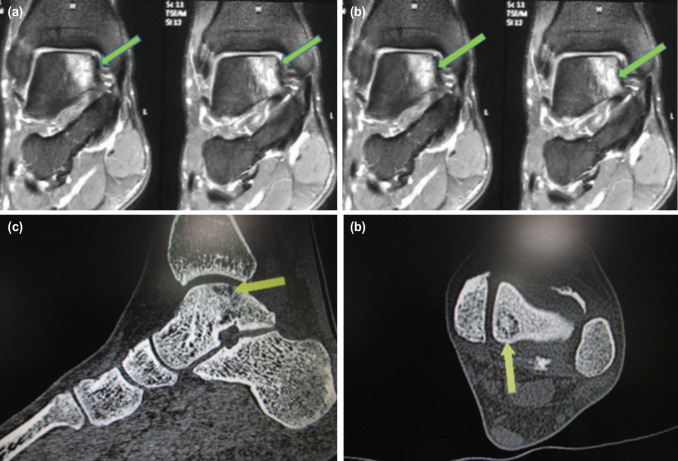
Imaging for diagnosis of OCD type V (a, b) magnetic resonance imaging; T1 and short-tau inversion-recovery (STIR) sequence respectively depicting the site of OCD; (c, d) CT scan images showing subchondral location of cyst). Green and yellows arrows depict the lesion.
